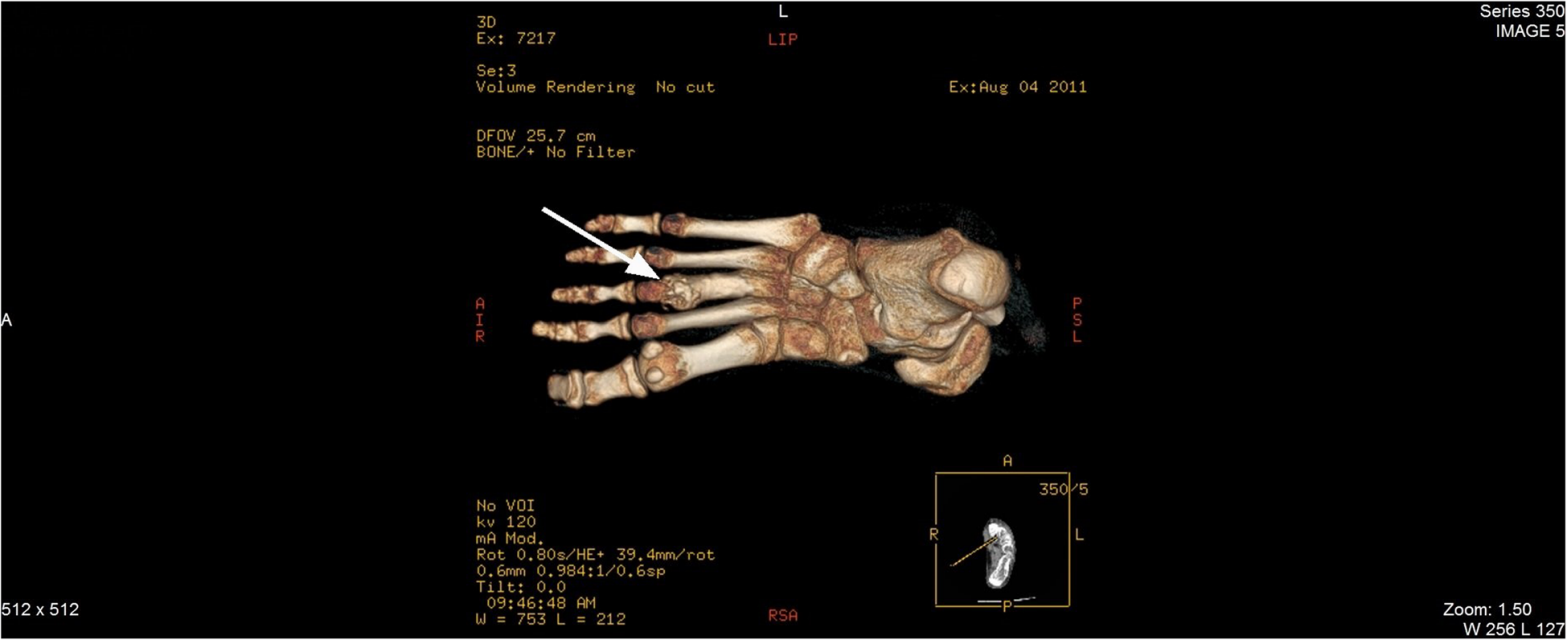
Three-dimentional reconstruction CT of the left foot. The destruction and sclerosis of third metatarsus bone diaphysis and distal metaphysis parts are indicated by white arrows
